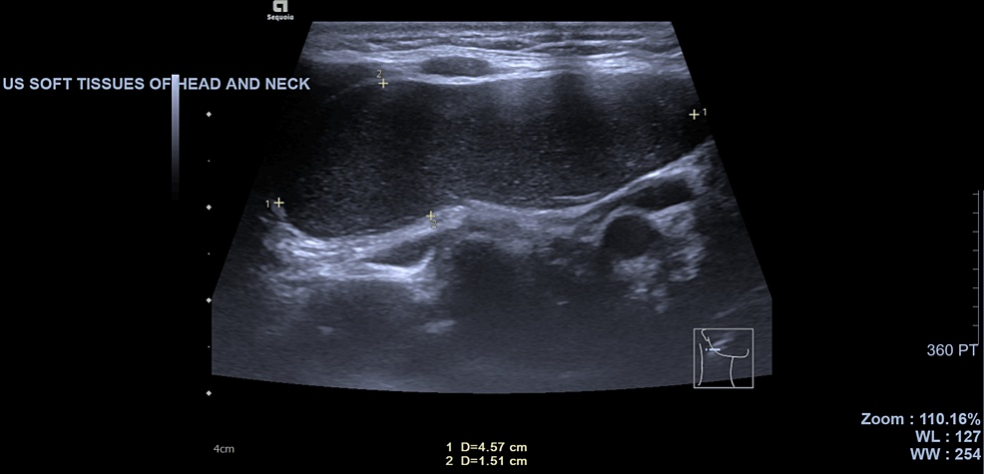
Ultrasonography of the right side of the neck and submandibular region showing the lesion's borders (marked with crosses).
